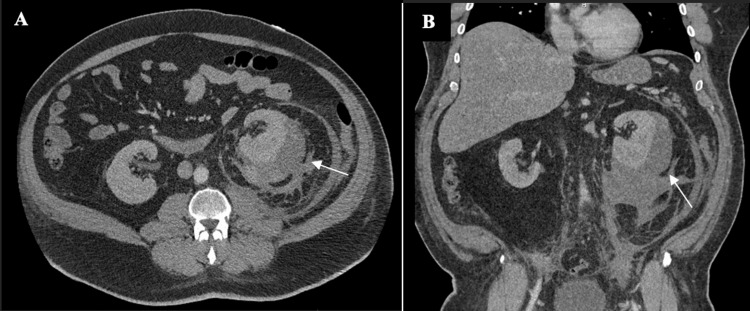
CT of the abdomen and pelvis with contrast. Subcapsular hematoma around the left kidney appears slightly increased in size (a). Pigtail drainage catheter is present within the subcapsular hematoma (b).
